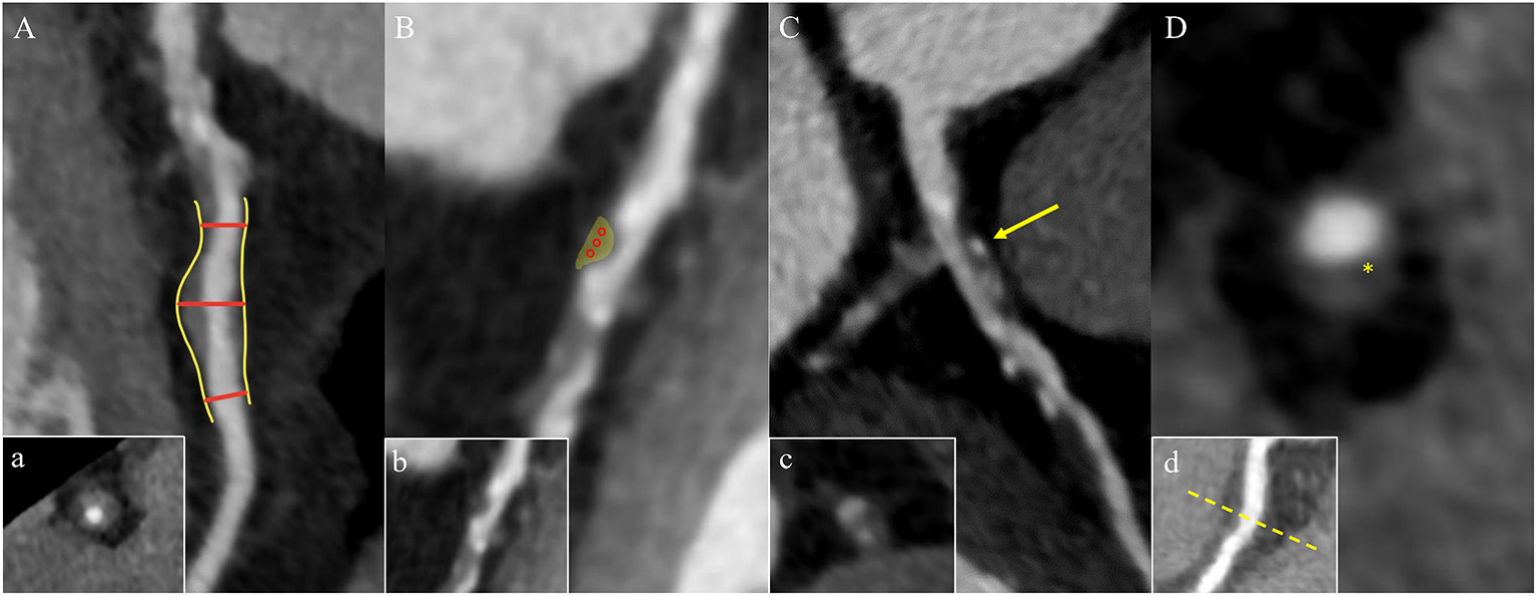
High-risk plaque characteristics on CCTA. (A) Positive remodeling of a non-calcified plaque in the proximal left anterior descending coronary artery. The two short red lines indicate the vessel diameters of the proximal and distal of the plaque (both 1.0 mm), and the long red line indicates the maximum vessel diameter in the middle of the plaque (1.6 mm). The remodeling index is 1.6. Picture a is the cross section of picture (A). (B) A low-attenuation plaque (yellow area) in the mid segment of the left anterior descending coronary artery with a mean CT attenuation value of 21 HU in the three regions of interest (red circles). Picture b is the original picture (B). (C) Spotty calcification of a partially calcified plaque surrounded by non-calcified components in the proximal left anterior descending coronary artery with a diameter <3 mm in all directions (yellow arrow). Picture c is the cross section of picture (C). (D) A napkin-ring sign plaque in the proximal right coronary artery. The yellow star shows the central area of the plaque with a low HU close to the lumen, which is surrounded by the peripheral edge of higher CT attenuation. Picture d is the multiplanar reconstructed image of picture (D). CCTA, coronary computed tomography angiography; HU, Hounsfield units.
